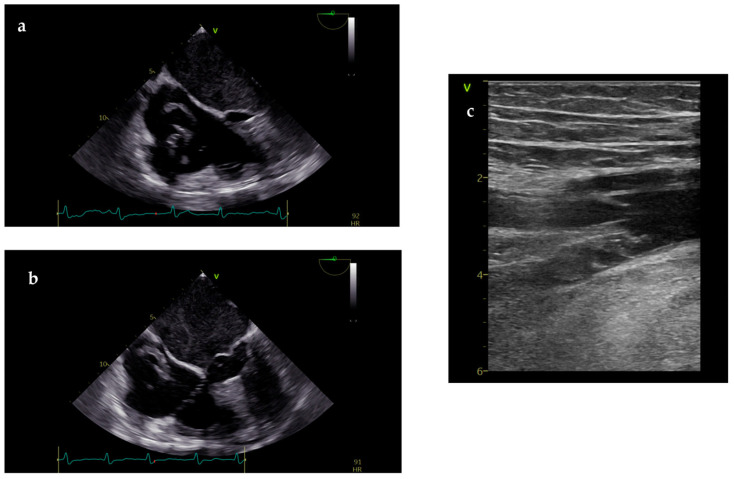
TEE performed during CPR in a patient with CA and non-shockable rhythm presentation, showing in-transit thrombus in right chambers suggesting PE as CA cause. Notably, the patient was affected by hypertrophic cardiomyopathy (a,b); vascular ultrasound performed in the same patient after cardiac resuscitation showed deep vein thrombosis involving superficial femoral vein (c).
